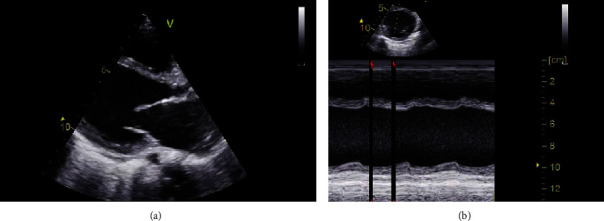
Echocardiographic evaluation at parasternal Log axis (a) and short axis (b) views shows left ventricular dilatation and decreased contractility.
